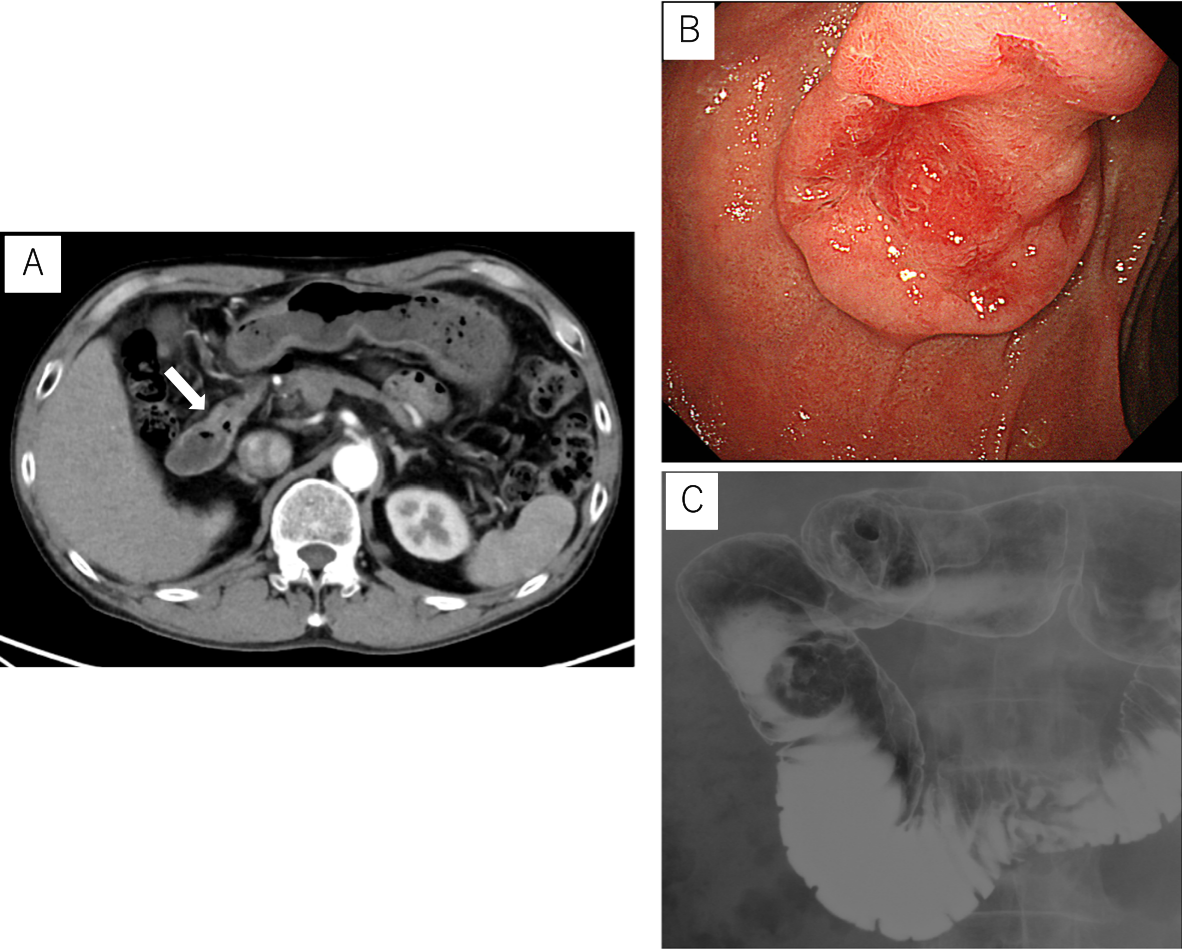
a An enhanced computed tomography revealed the thickened wall of the duodenum without invasion outside the wall, nor lymph node swelling (arrow). b Gastrointestinal endoscopy showed a type 2 tumor, measuring about 25 mm in diameter at the second portion of the duodenum. c An upper gastrointestinal image revealed a clearly demarcated filling defect in the second portion of the duodenum
